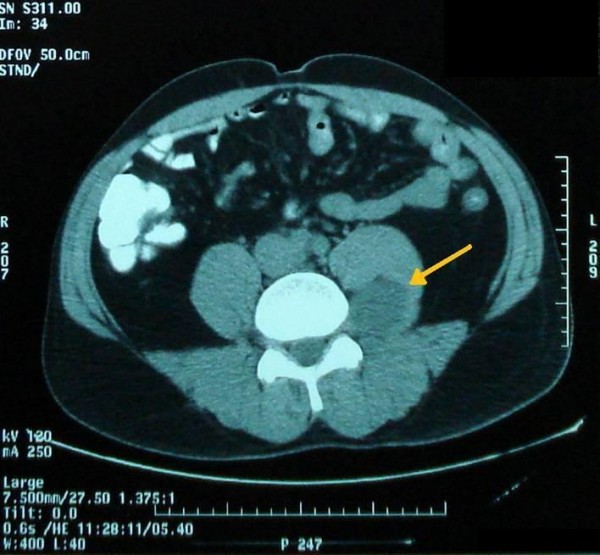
CT showing a 5 cm mass in the left lumbar paraspinal region, indicated by the arrow.
